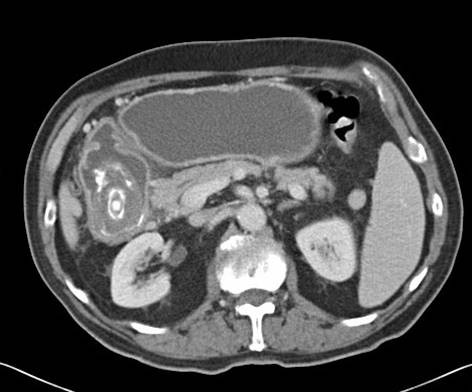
Abdominal CT demonstrating a calcified mass in duodenum.
